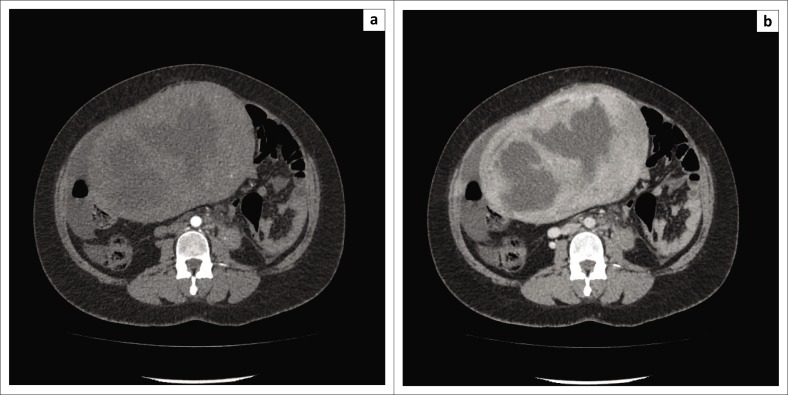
Axial computed tomography of the abdomen during (a) arterial and (b) portal venous phases showing a large centrally hypodense mid-abdominal mass with a thick enhancing wall and septations.
